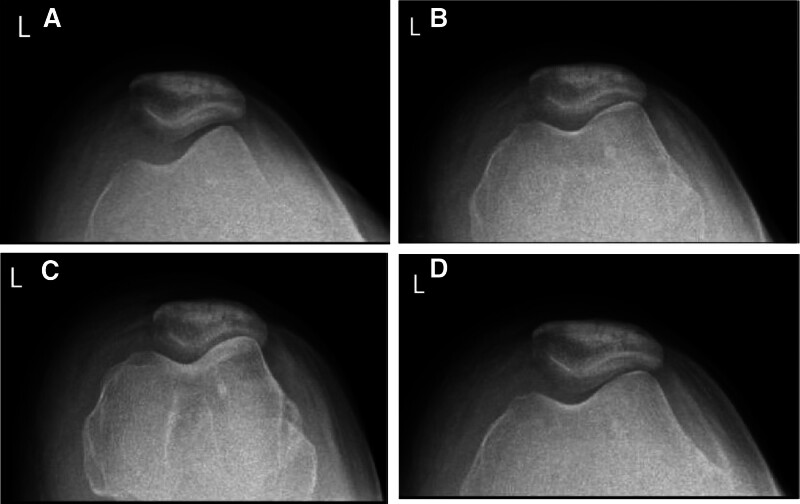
Preoperative standing full-length anteroposterior radiograph of the lower limbs demonstrating significant valgus malalignment (HKA: 175.42°, MAD: 16.14 mm lateral deviation). MAD = mechanical axis deviation, HKA = Hip-knee-ankle angle.
